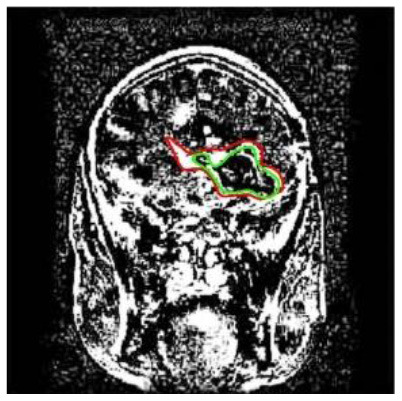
Patient 5 is a radiation necrosis (RN) patient with malignant rhabdoid and a posttreatment entity (43). It occurred 3 months after radiotherapy and gave the effect of capillary damage. Patient 4 demonstrated a pseudoresponse after receiving bevacizumab for the past 7 months. Axial, coronal and sagittal slices of brain MRI sequences of original and current images as well as the subtracted image were shown. These result slices of registration subtract images are colour coded to label signal changes (volume and diameter) over time. Green denotes new or progressive tumours, and red denotes the original tumour.
